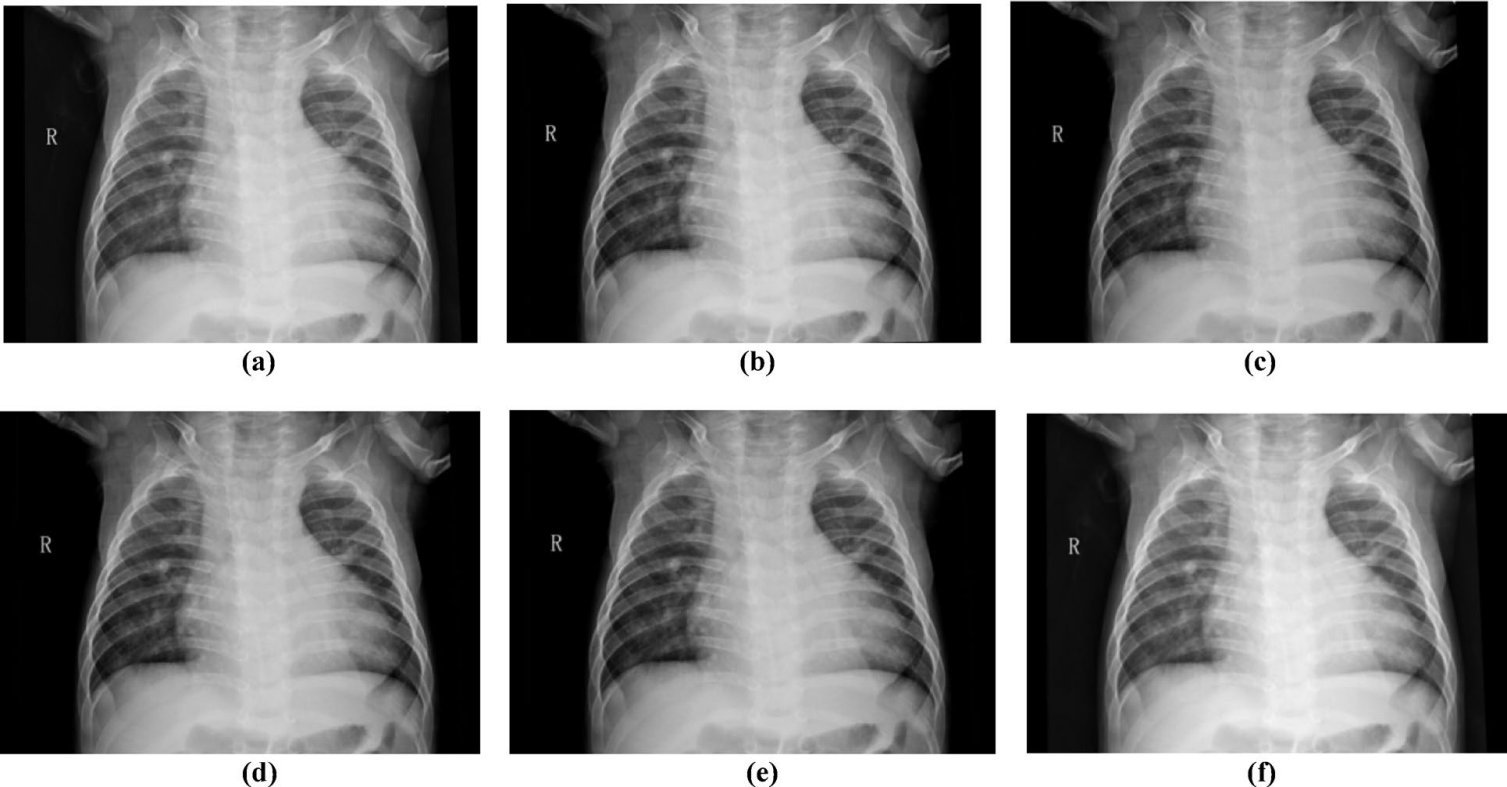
Visual comparison of X-ray images under different denoising methods. (a) Original noisy image, denoised image using(b) Gaussian Pyramid, (c) wavelet-Haar, (d) wavelet-coif4, (e) wavelet-db4, and (f) wavelet-sym4.
